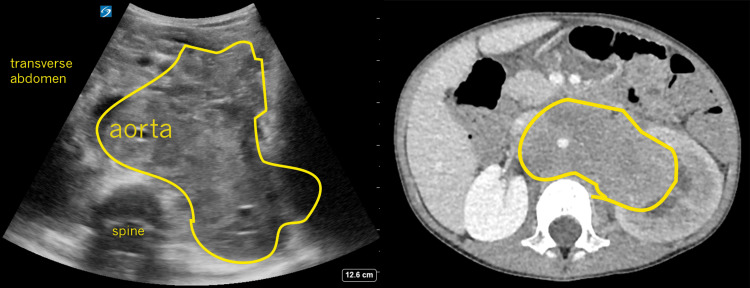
Transverse Abdomen Side-by-Side Views of Bedside Ultrasound and CT Abdomen/Pelvis Bedside ultrasound image (left) of the aorta encased in the mass arising likely from the right side which can be seen better on the CT scan (right) with the aorta lumen enhanced by contrast.
